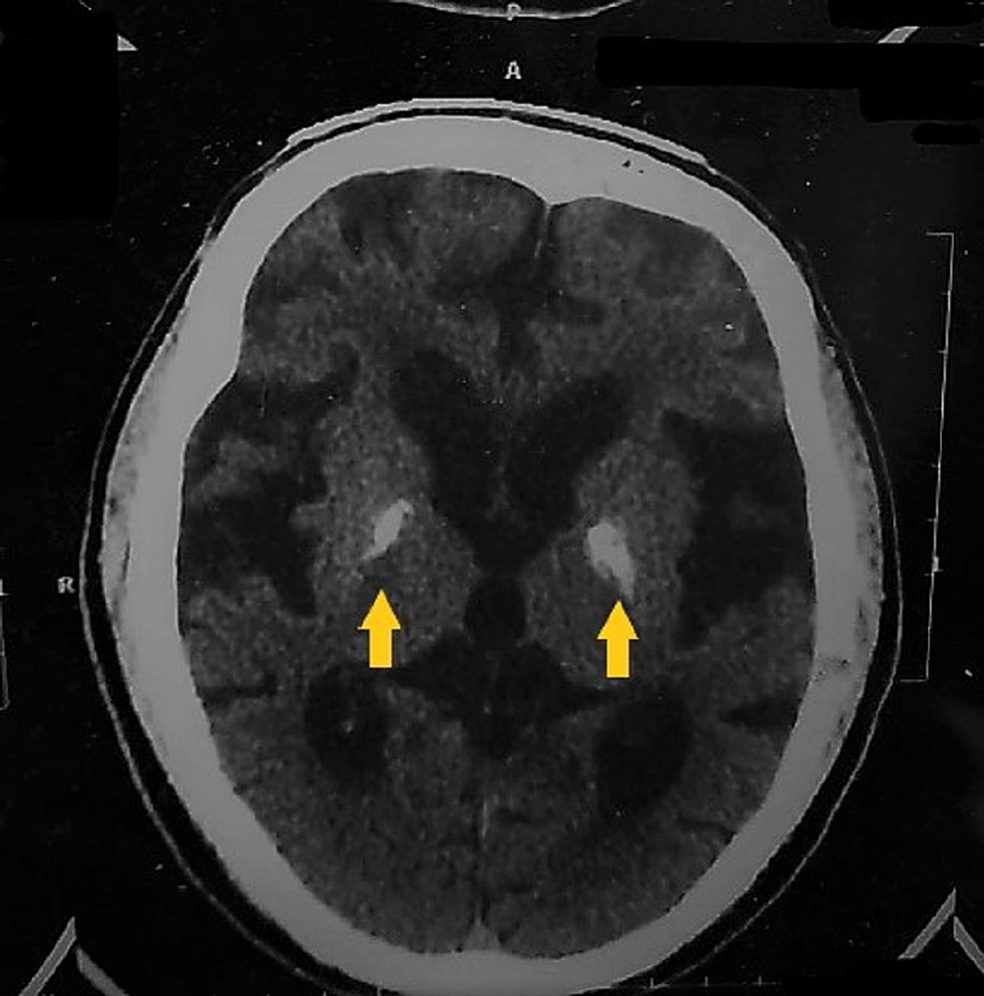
CT Brain showing bilateral basal ganglia whitish calcification (indicated by the colored arrows).
